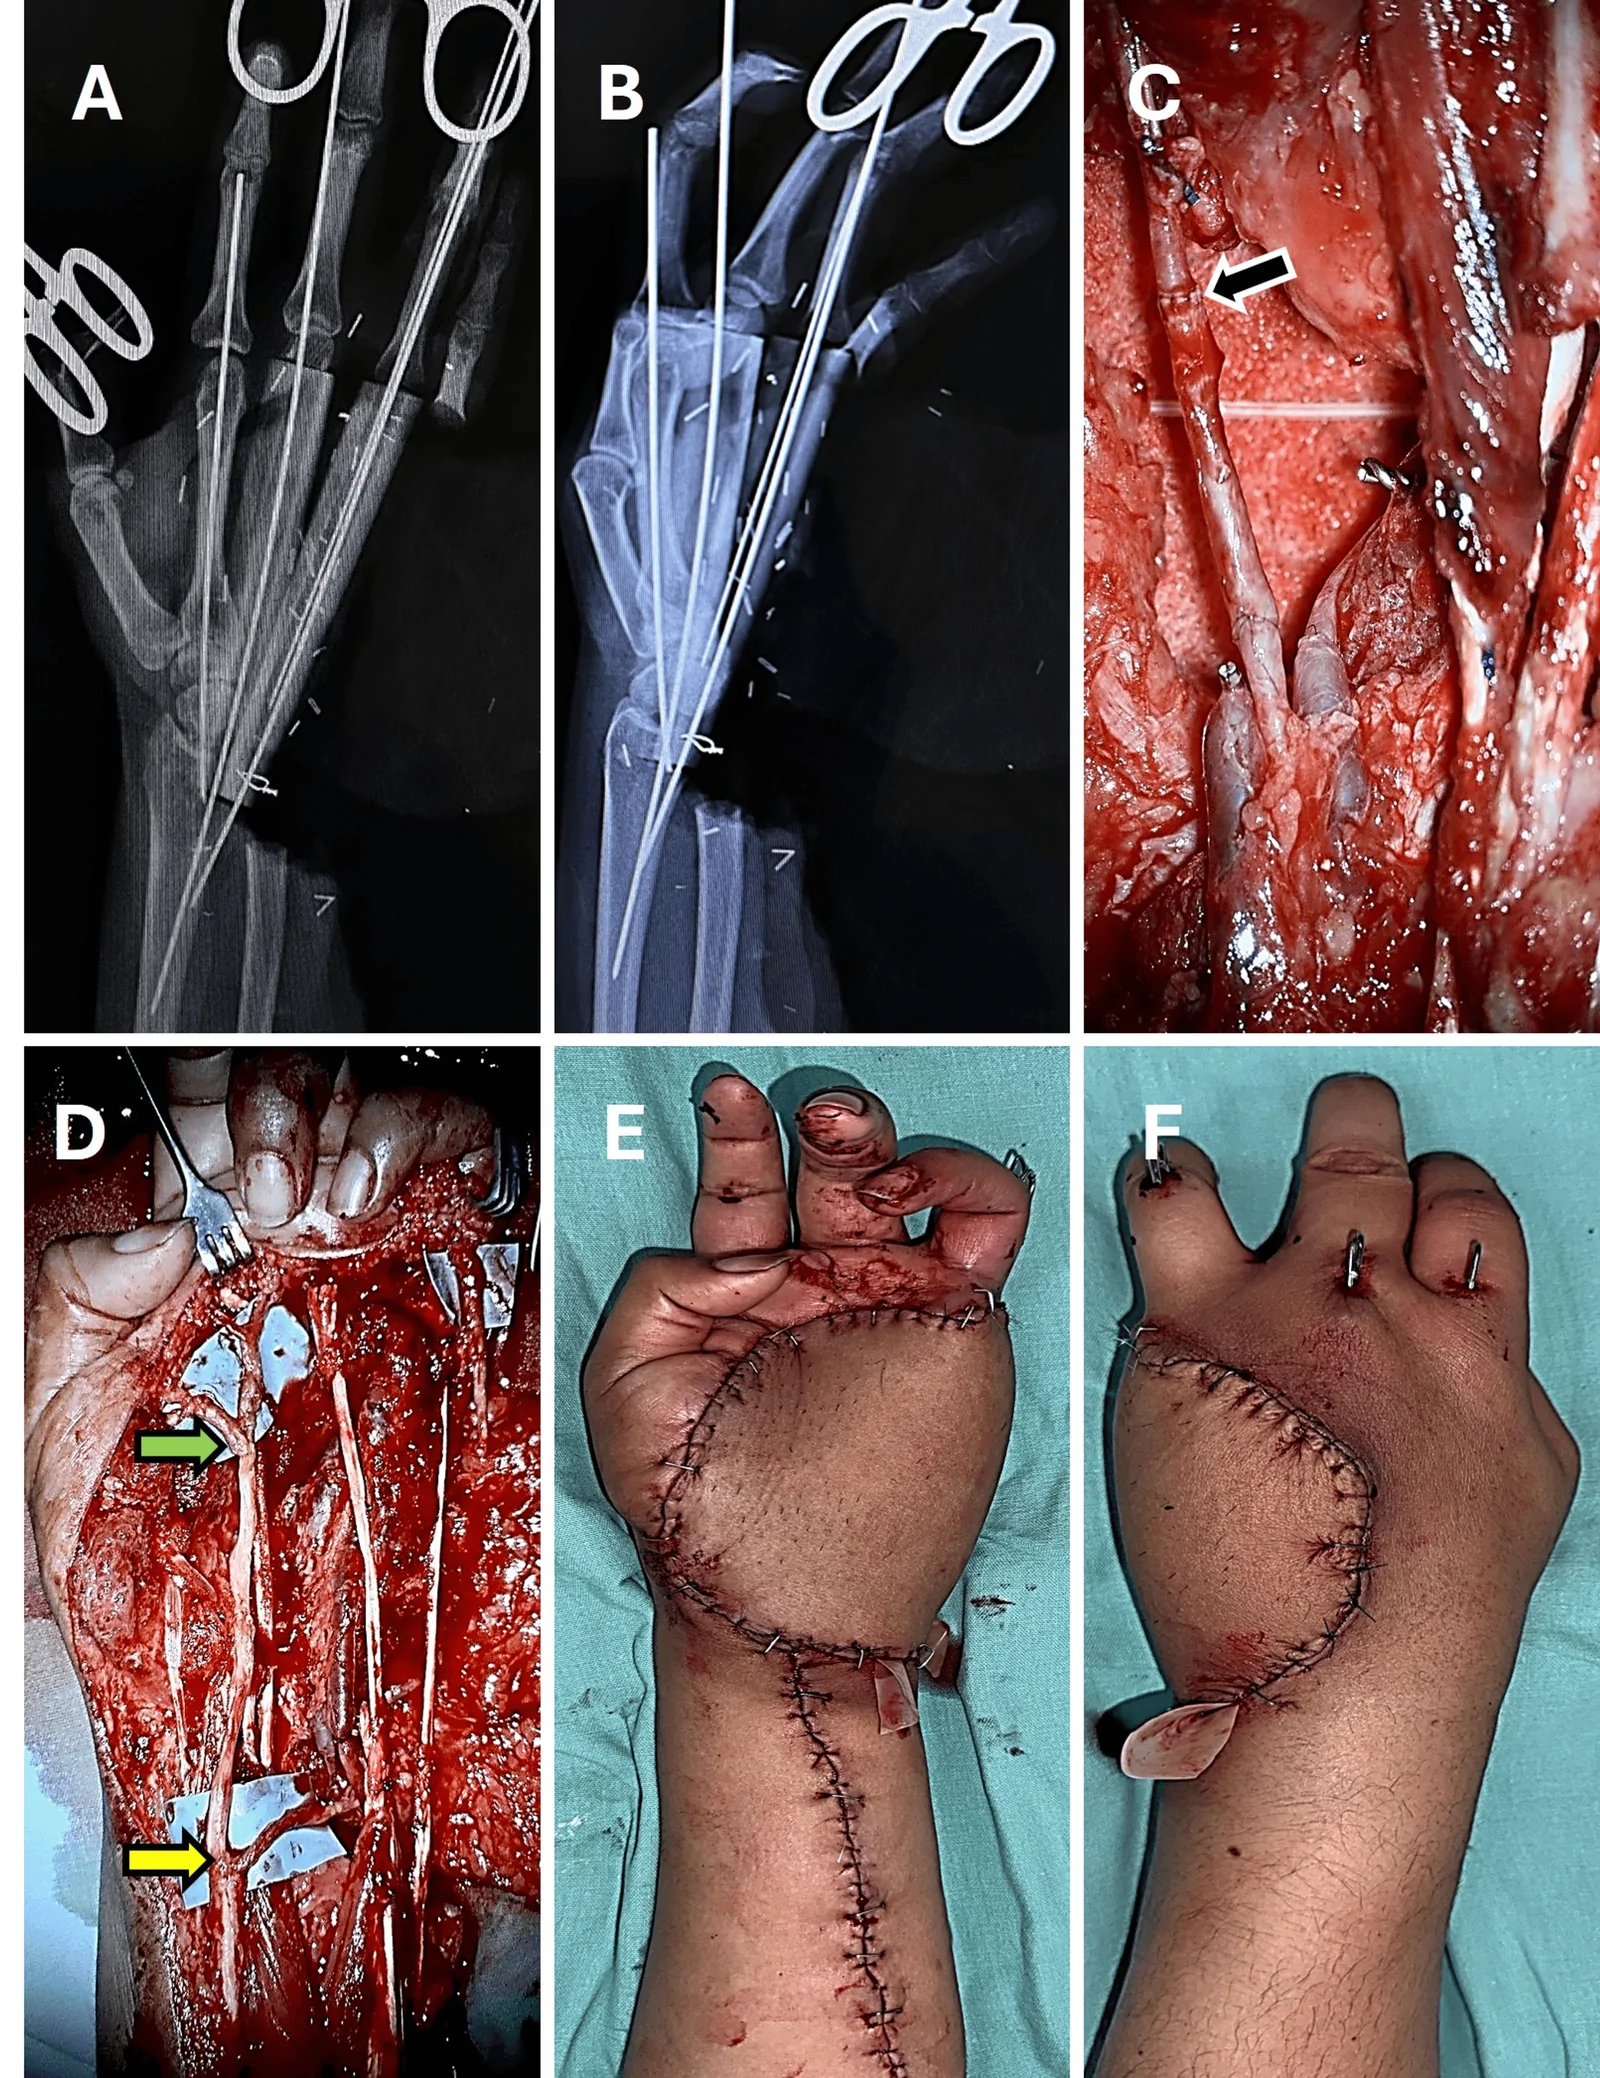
Hand reconstruction surgery after tumor resection. A) Intraoperative X-ray, showing the positioning of the 0.62 Kirschner wires in anteroposterior view and B) oblique view. C) Vascular microanastomosis, end-to-end, from the ulnar artery to the peroneal artery (black arrow). D) Intraoperative image of the hand reconstruction, showing tenorrhaphy with superficial tendon grafts to the deep flexors (green arrow) and nerve coaptations of the median nerve (yellow arrow) with an ulnar nerve graft to the digital nerves of the first, second, and third fingers and the ulnar collateral nerve of the fourth finger. E) Final postoperative image of the hand reconstruction in anterior view and F) posterior view.
